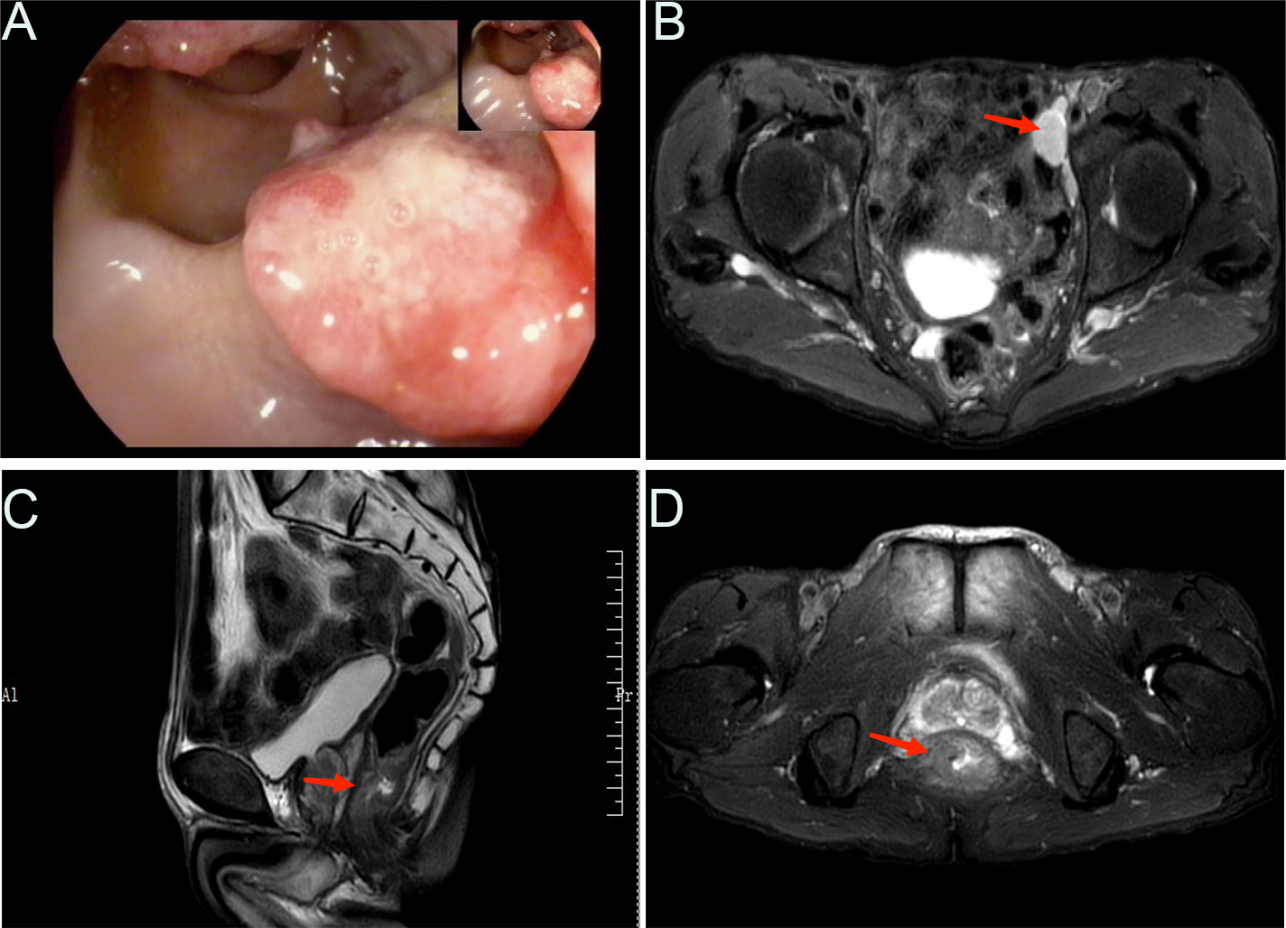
Radiological examination findings. (A) Representative image showing the colonoscopic view of the colon. (B) MRI findings, with an arrow indicating an enlarged lymph node adjacent to the left iliac artery. MRI findings of a rectal mass in both sagittal (C) and coronal (D) views, as indicated by the arrows.
